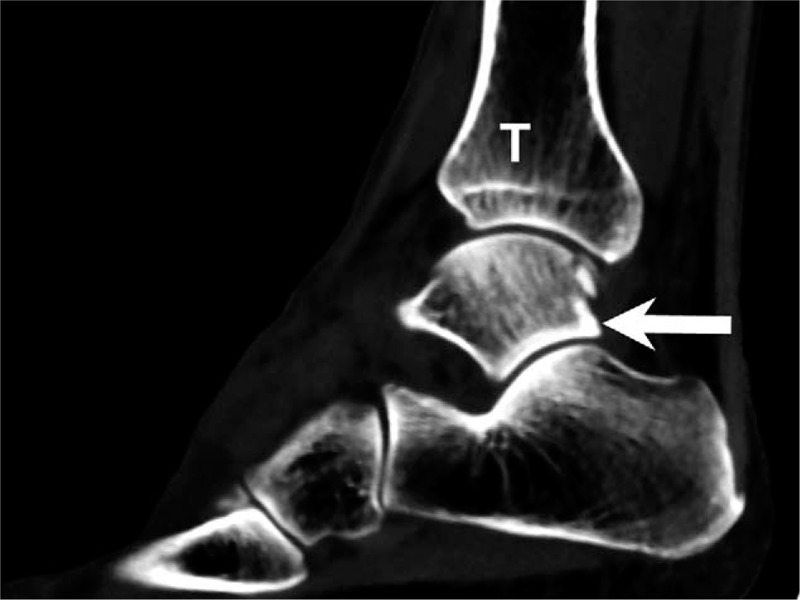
Computed tomography sagittal view of the posterior tubercle fracture of the talus. Arrow = talus, F = fibula, T = tibia.
